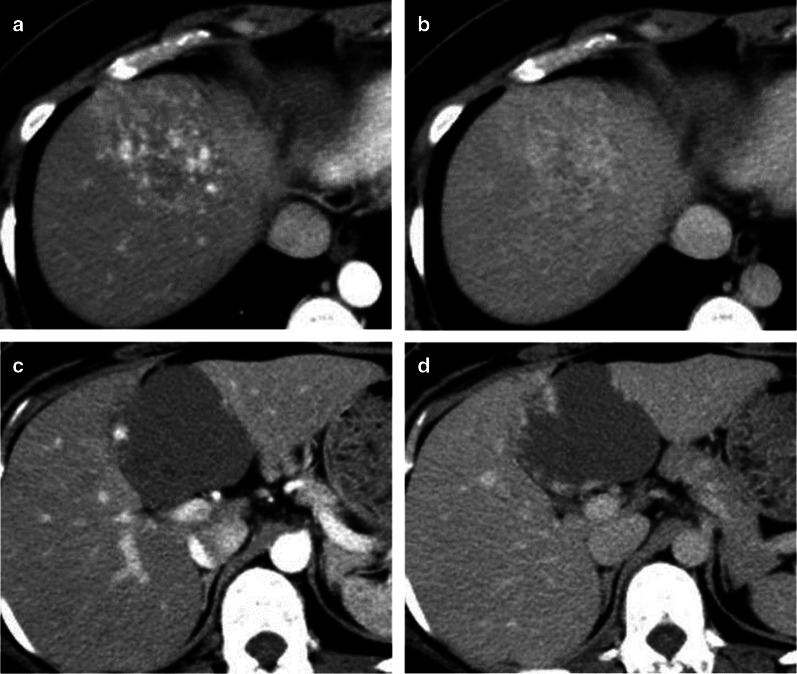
Enhanced CT findings. a, b Multiple ill-defined spots are heterogeneously enhanced in liver segment IVa. c, d An enhanced abdominal CT scan shows peripheral enhancement in the arterial phase (c) and fill-in contrast in the late phase (d) in liver segment IVb
